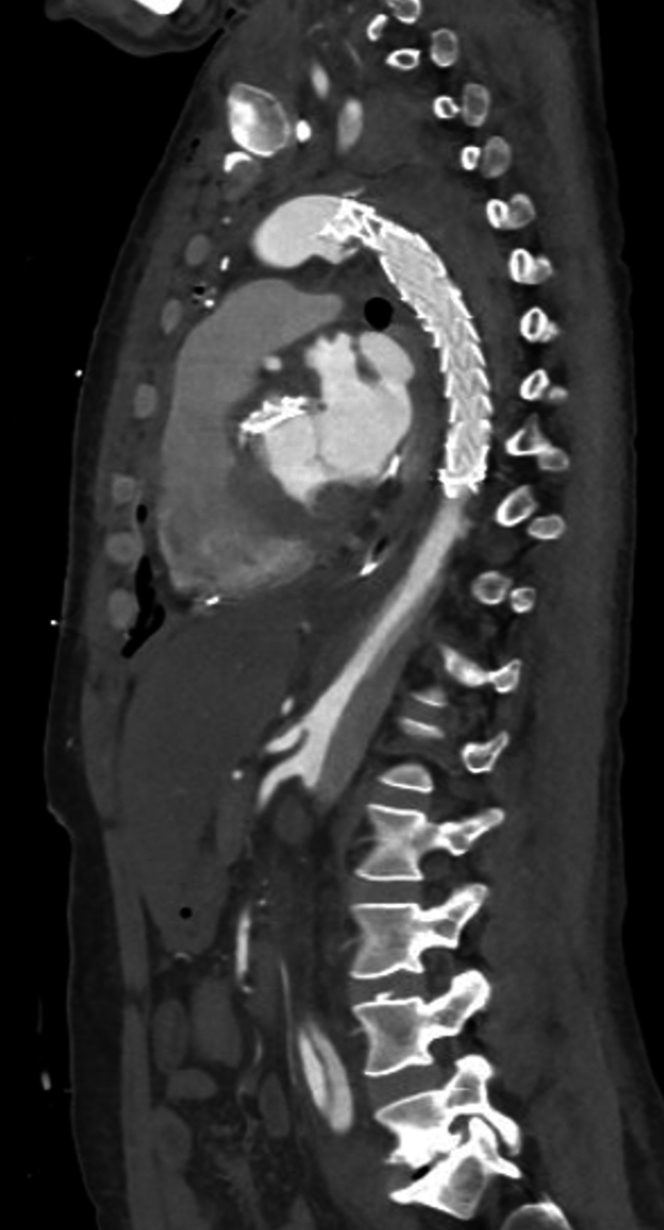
Sagittal Computed Tomography Showing the Aorta After Aortic Root Replacement Surgery
